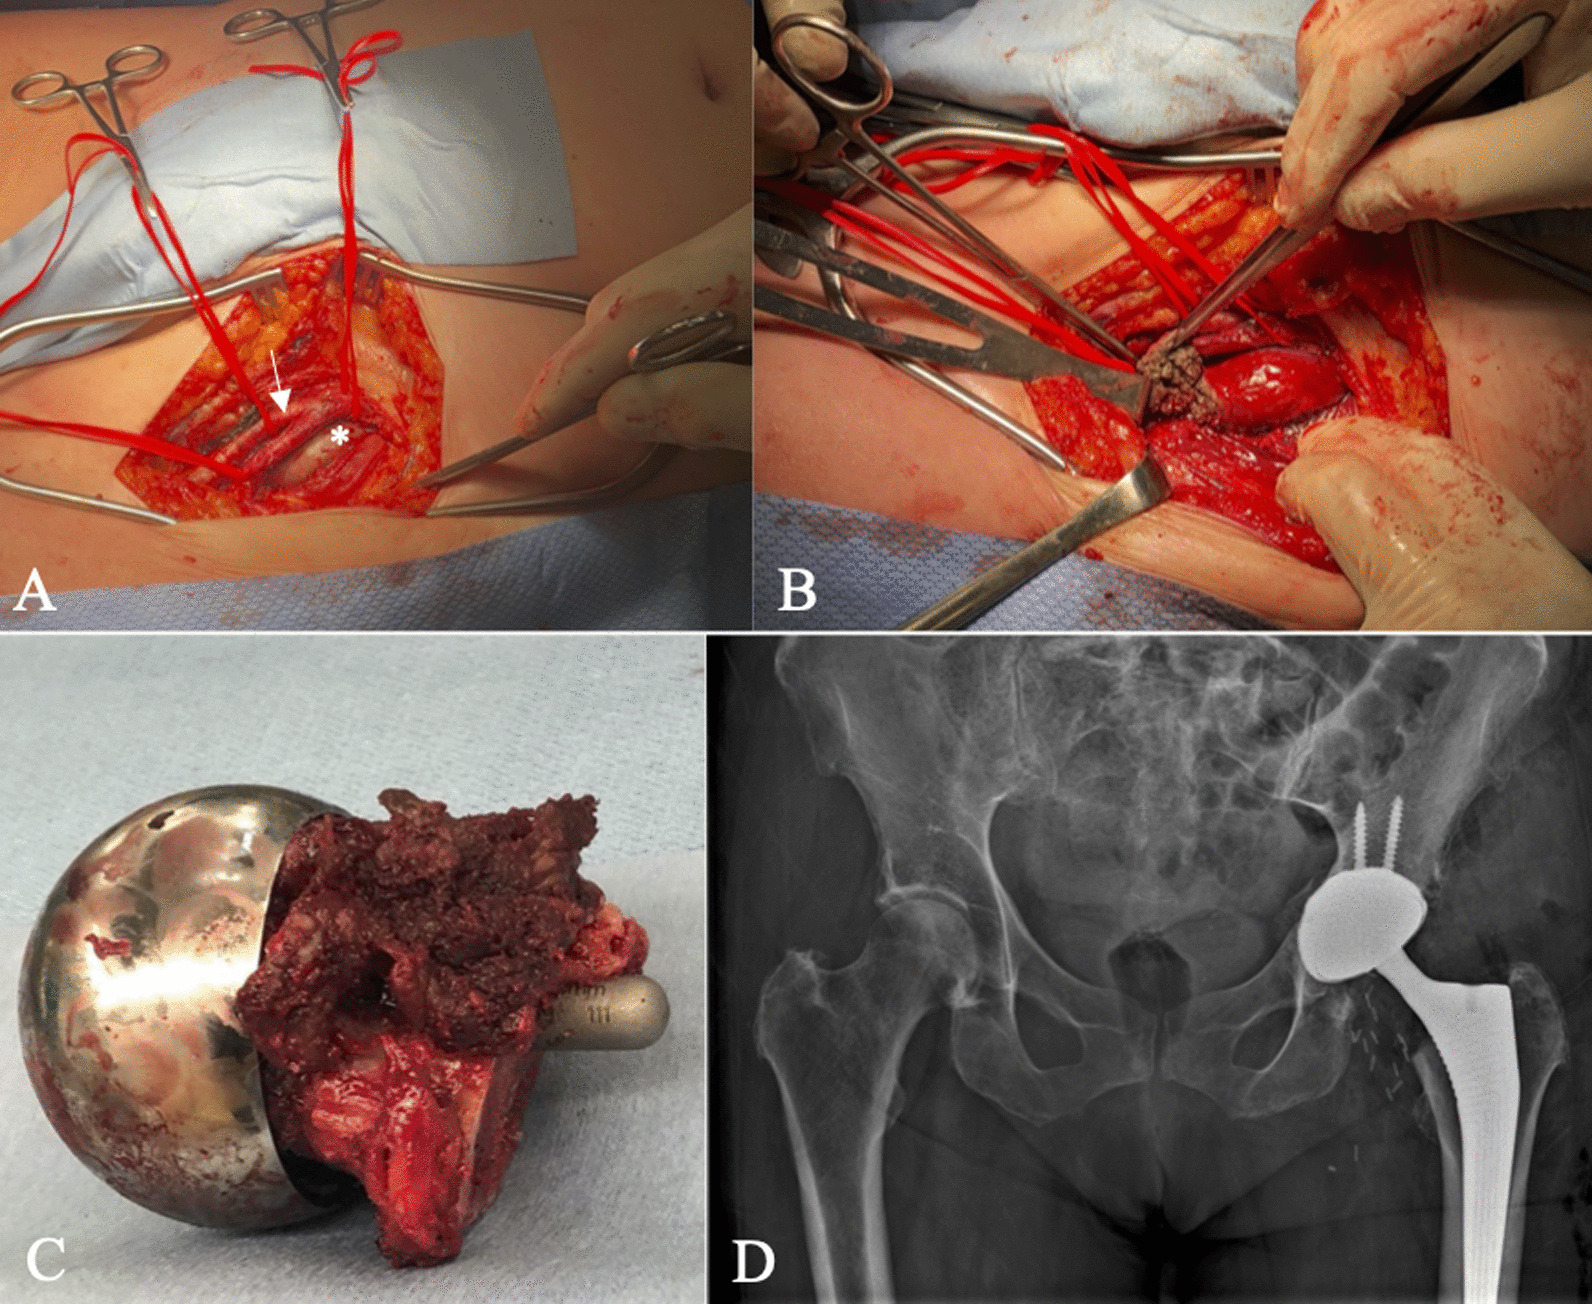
Intraoperative images and immediate postoperative radiograph demonstrating A anterior pseudotumor (asterisk) dissection from the posterior aspect of the superficial femoral artery (arrow), profundal femoris, and femoral nerve; B the pseudotumor was ultimately taken out piecemeal to excise it safely; C pseudotumor was adherent to the anterior femoral neck and resulted in significant bone erosion; D immediate postoperative AP pelvis X-ray of the conversion to total hip arthroplasty
